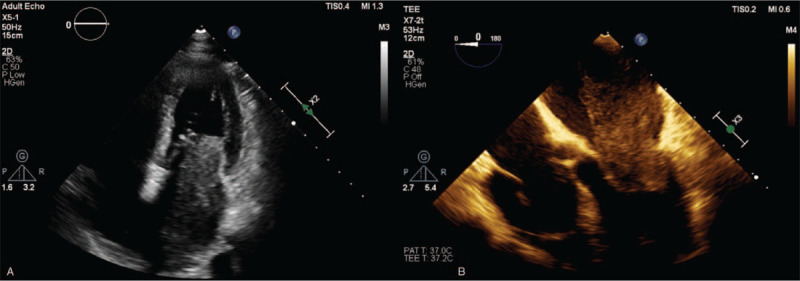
Compared with Routine TTE(A), TEE(B) was able to completely present the size, irregular shape, markedly heterogeneous internal echoes, broad base, involving the left atrial wall and the posterior leaflet of the mitral valve with a wide range and unclear boundary and adhesion of the mass in the same section (Apical four-chamber heart section).
